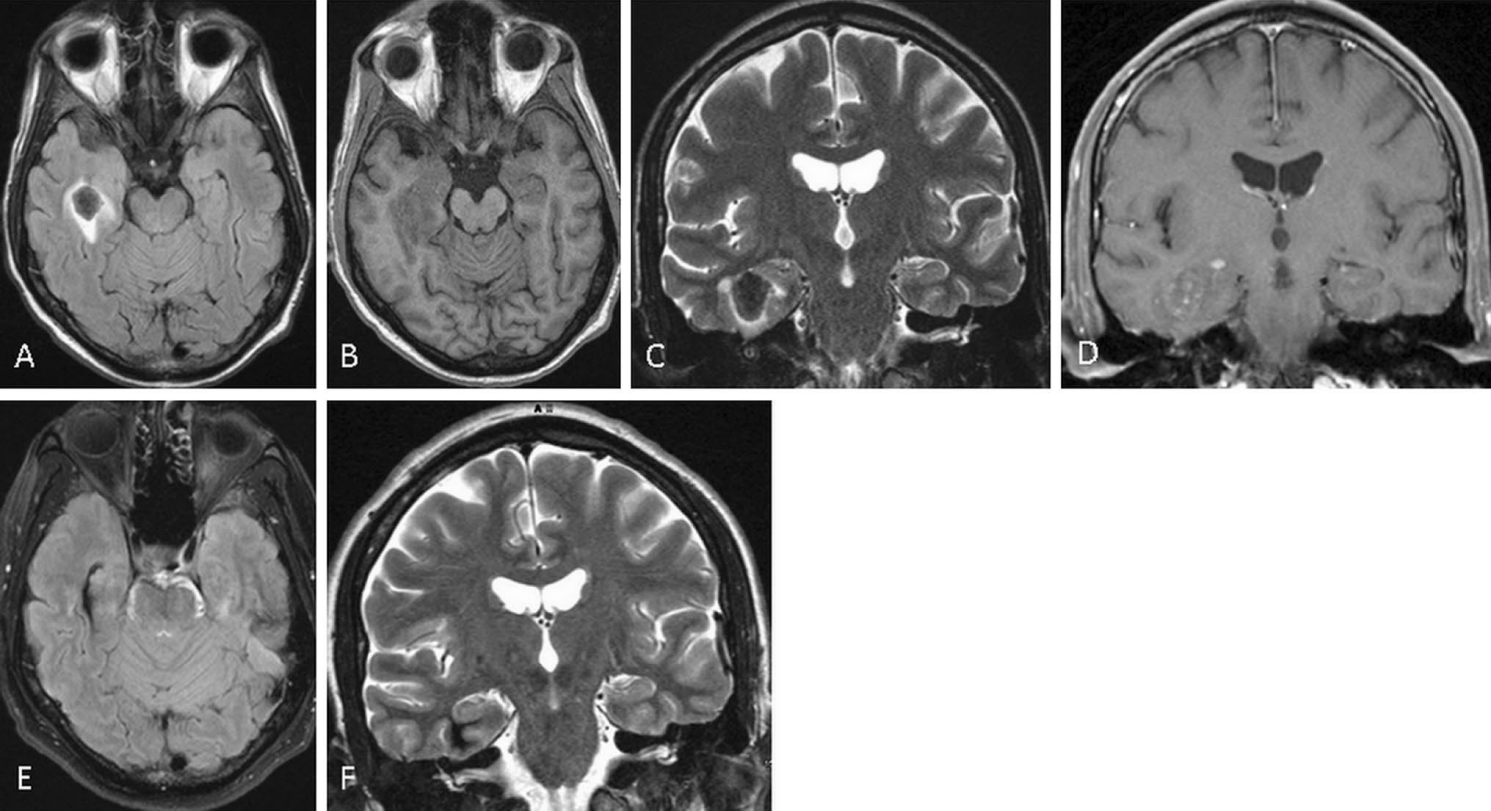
On the initial MR exam, there was a 2-cm FLAIR hypointense (a), T1WI isointense (b), T2 hypointense (c) lesion in the right temporal lobe adjacent to the temporal horn, with perilesional edema and patchy contrast enhancement (d). At the 6-month follow-up MR scan, there was only a small scar with hemosiderin deposits (e, f)
